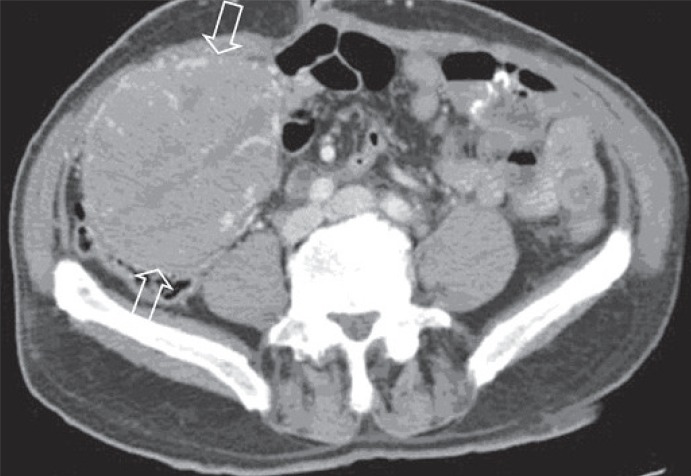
Abdominopelvic helical Computed Tomography, performed 8-months after surgery, revealed a voluminous mass in the right iliac fossa (arrow). After intravenous contrast material administration the masses showed heterogeneous enhancement and well-defined margins.
